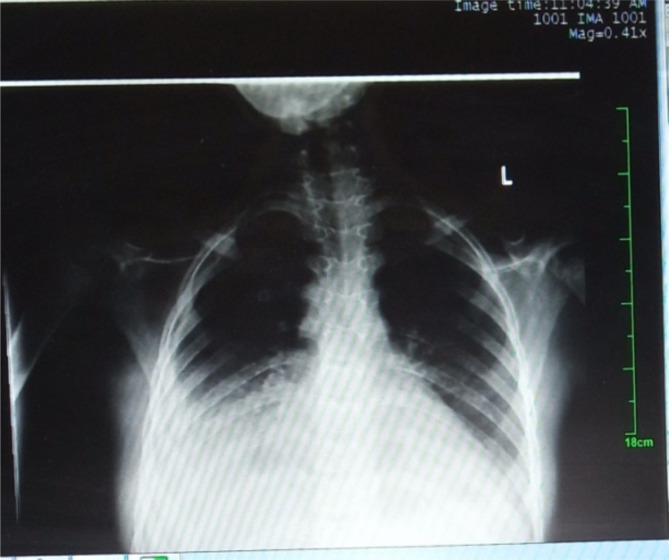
chest x-ray of the 10 year old girl with MIS-C : bilateral pleural effusion with collapsed basal lung segments (more on the right), February, 2021.
